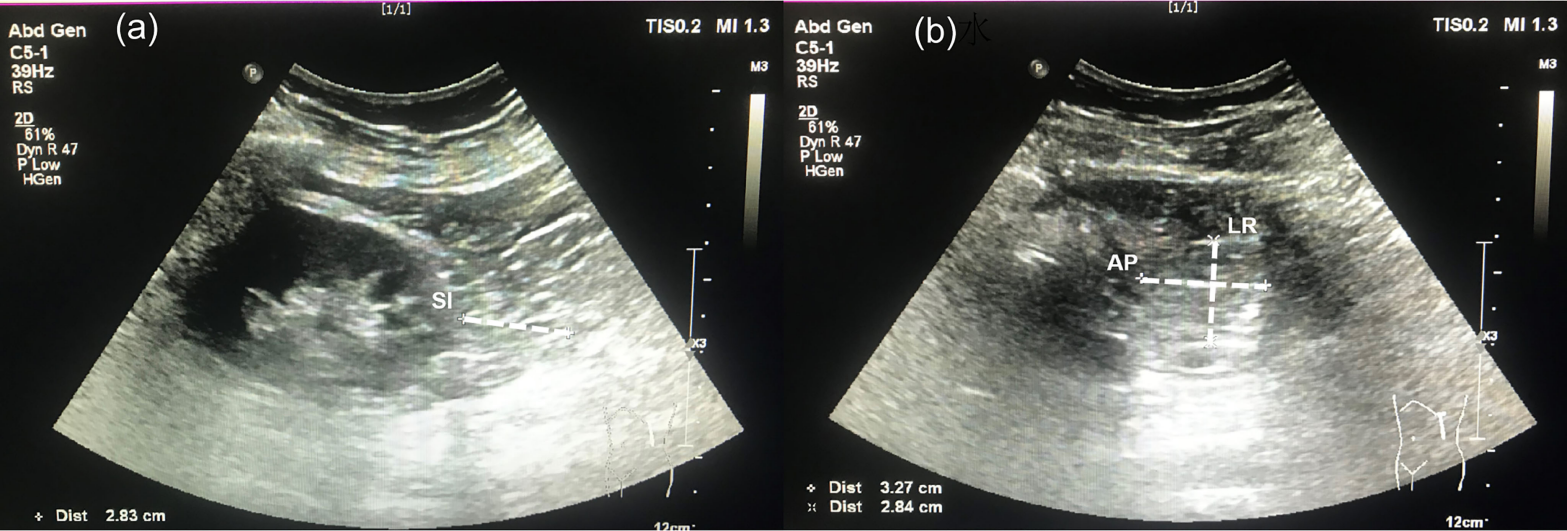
Ultrasonographic measurement for perirenal fat volume. (A) A long-view of the inferior perirenal fat. Superior-inferior (SI) diameter was defined as the maximal thickness between the fibrous membrane to the renal fascia. (B) A short-view of the inferior perirenal fat. The left-right (LR) diameter and anterior-posterior (AP) diameter were measured through the maximal short-axis view of the inferior perirenal fat.
